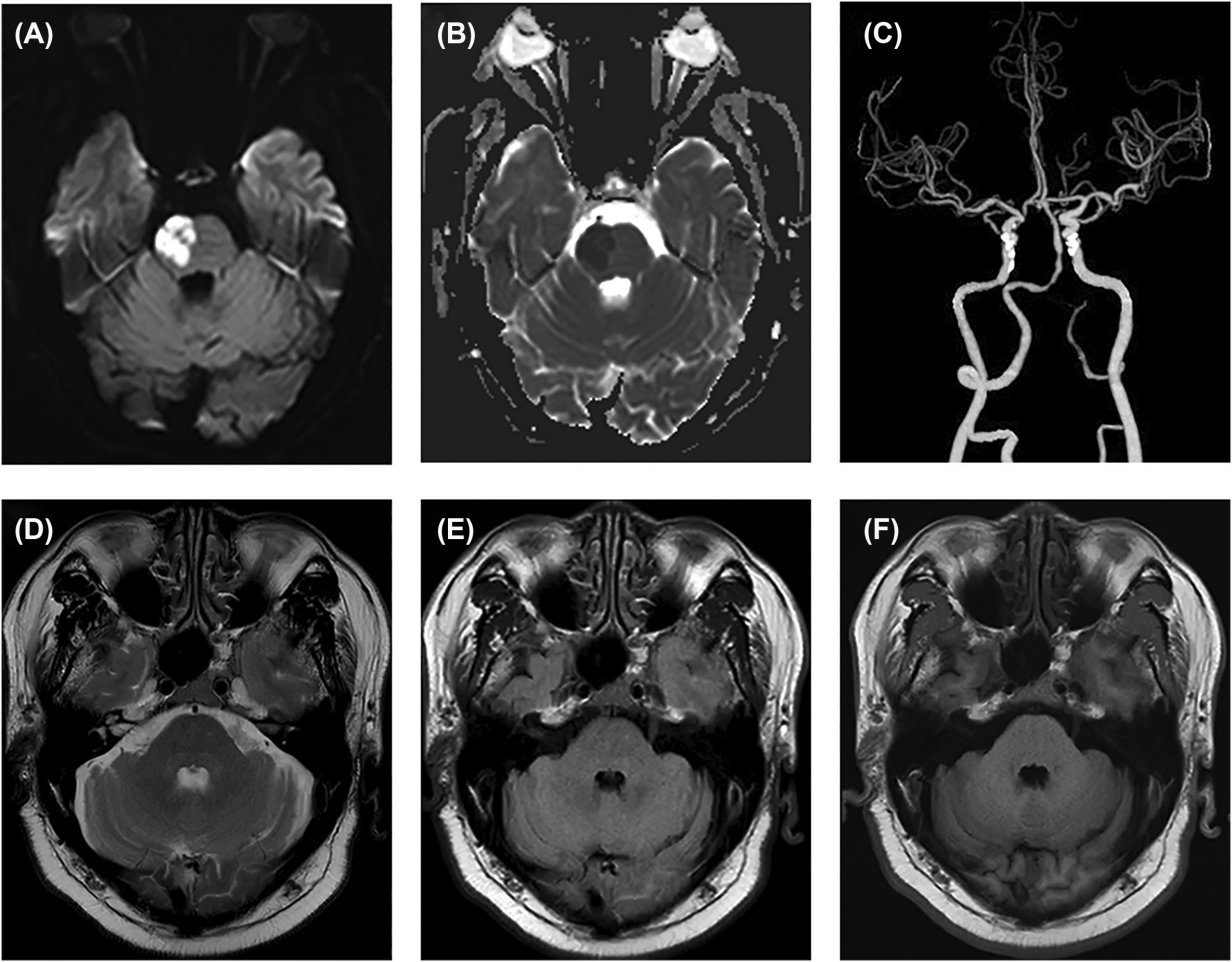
The initial MRI examination of patient 2 revealed acute right pontine infarction. (A, B) High diffusion‐weighted imaging (DWI) signal intensity, and low apparent diffusion coefficient signal intensity, in the right pons. (C) Computed tomography angiography (CTA) showed multifocal stenosis of left vertebral artery and basilar artery. (D–F) There were no abnormal signals in the bilateral middle cerebellar peduncles.
